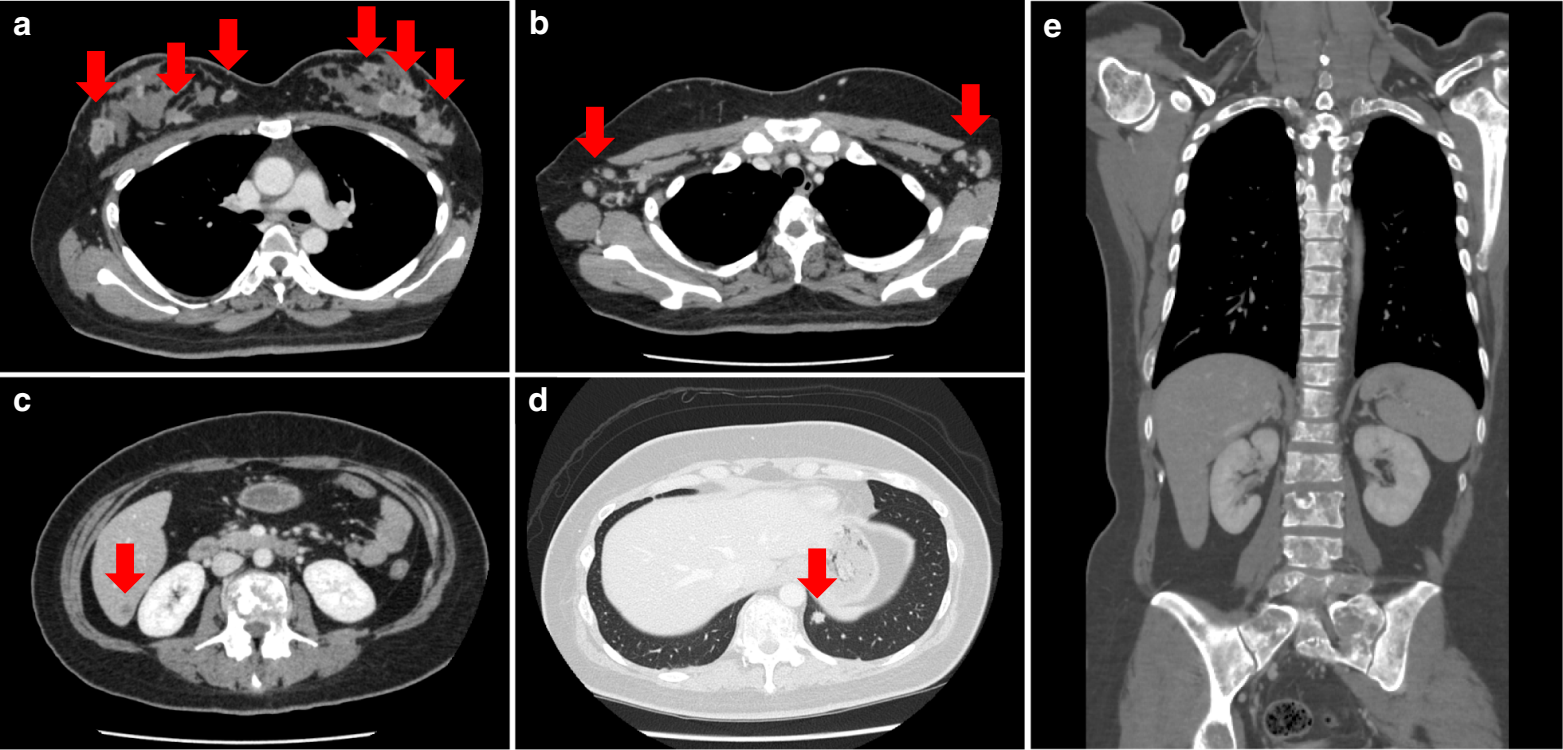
Contrast-enhanced CT images. a Contrast-enhanced masses occur frequently in bilateral breasts (arrows). b Metastasis to bilateral axillary lymph nodes (arrows). c Metastasis in the S7 area of the liver (arrow). d Metastasis to the lower lobe of the left lung (arrow). e Metastasis on all observable vertebral bodies
